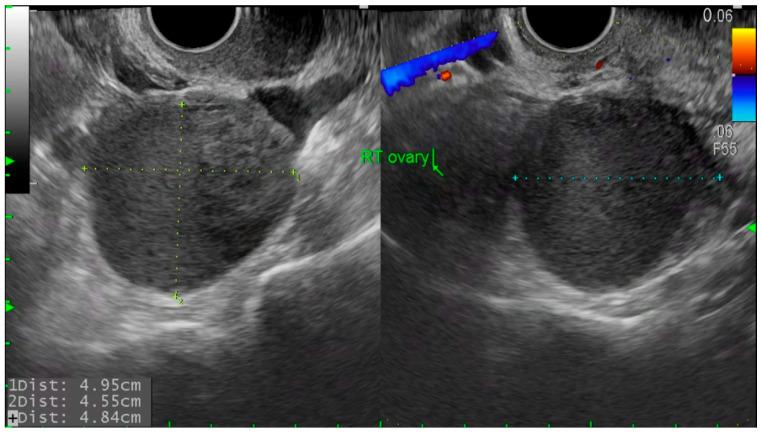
Transvaginal ultrasound image of recurrent ovarian endometrioma. Round, thick-walled cyst with homogenous low echogenic content and scattered internal echoes, measuring over 2 cm in diameter.
